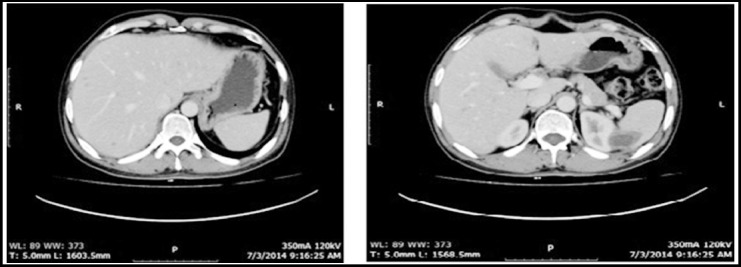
(A and B): CT neck, chest, abdomen and pelvis with contrast showed interval development of multiple focal hepatic and splenic hypodensities.
